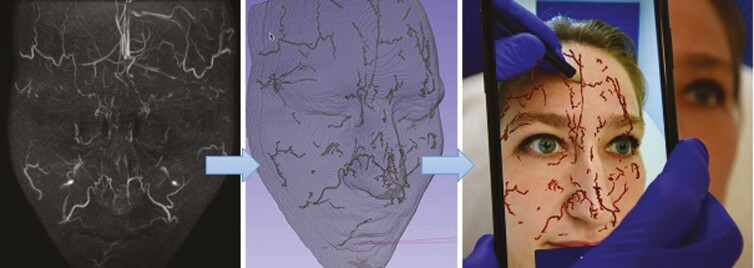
Magnetic resonance angiography findings and augmented reality (AR) visualization in a 26-year-old female. (A) Infrared thermally enhanced MRI without contrast. Frontal view of a maximum intensity projection (MIP) from the 3-dimensional time-of-flight multiple overlapping thin slab acquisition (3D-TOF MOTSA). (B) Segmentation of subcutaneous arteries. 3D image after isolation of the arteries and conversion to a 3D volume. (C) Visualization of subcutaneous arteries through AR and projection on the face of the patient with a smartphone camera.
